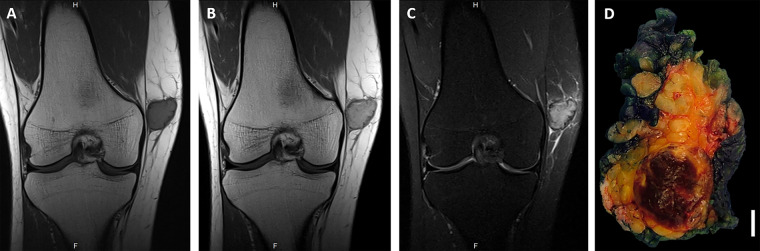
Representative images of the radiological (A–C) and gross (D) features of *HMGA2-NCOR2* fusion-positive giant cell tumors (Case 2). Coronal T1-weighted (T1W) pre- (A) and post-contrast (B) and proton-density (C) MR images show an enhancing deep subcutaneous mass that abuts the peripheral fascia at the distal medial aspect of the right thigh. The slight hyperintensity of the tumor relative to muscle on pre-contrast T1W images and the peripheral low signal on all sequences likely represent its hemorrhagic content. Mild reticular edema surrounds the lesion. The epicenter of the tumor is in the subcutaneous tissue with well-circumscribed borders and tan to brown lobulated cut-surface (D).
